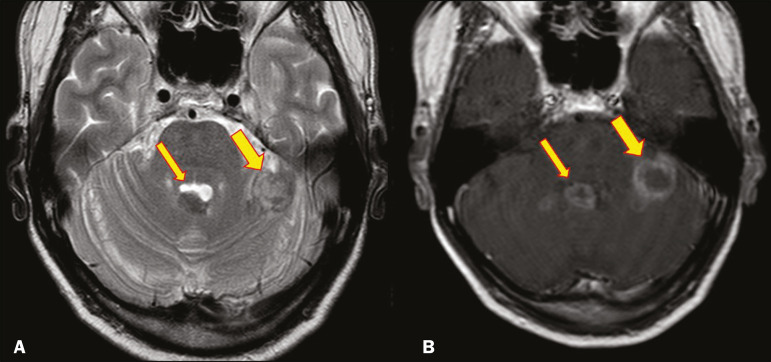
A 53-year-old alcoholic male patient, from a rural area, with a history of chewing grass. MRI showed granulomas in the CNS, suggestive of paracoccidioidomycosis. A: T2-weighted image showing lesions with heterogeneous low signal intensity in the cerebellum (arrows). B: Contrastenhanced T1-weighted image showing hypointense lesions with peripheral ring enhancement in the cerebellum (arrows).
